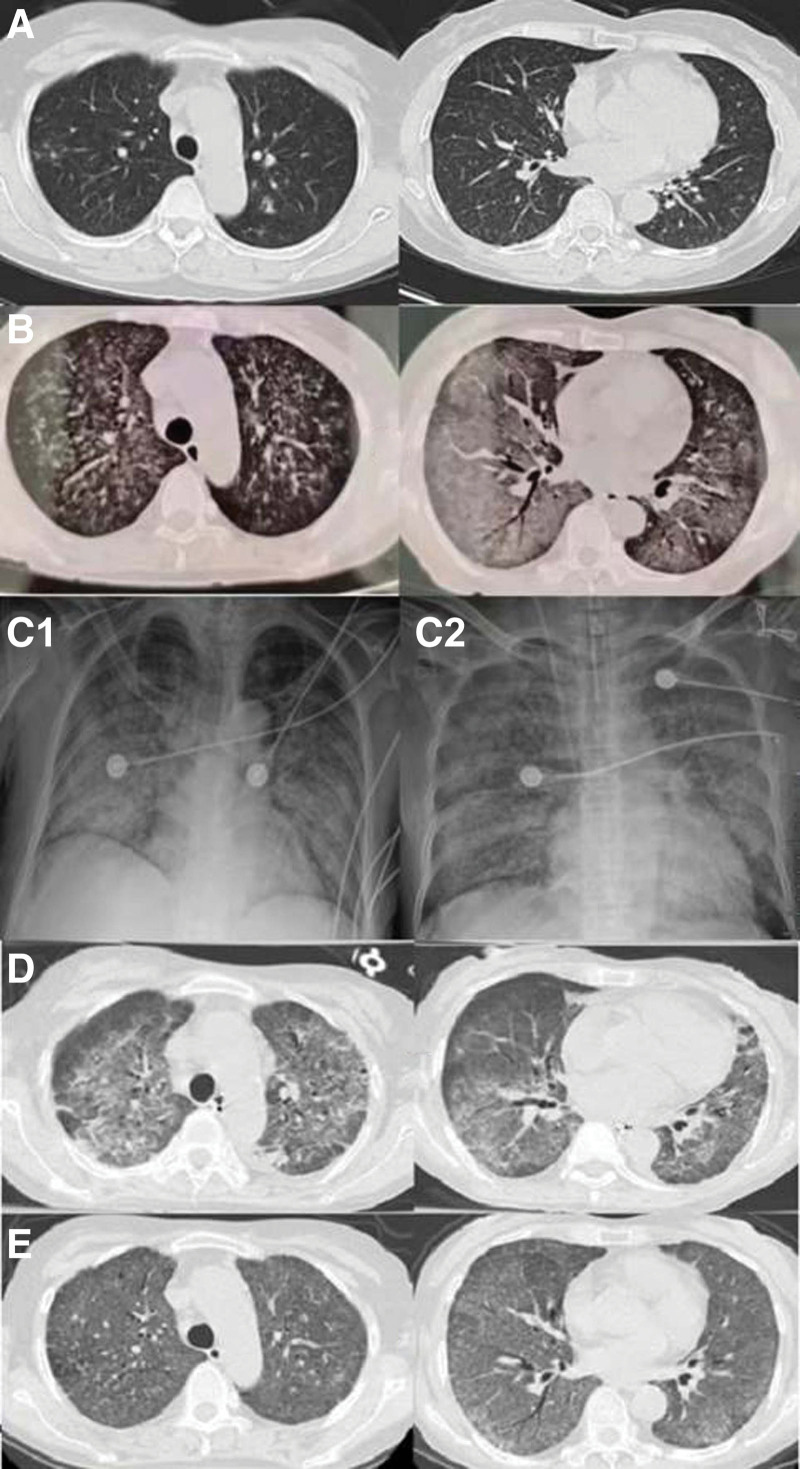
Chest computed tomography scan. (A) Bilateral pulmonary nodules during the first clinic visit. (B) Bilateral patchy infiltrations, consolidations, and ground-glass shadows 19 days after initiating antituberculosis therapy. (C) Right, at the intensive care unit admission, chest X-ray showed bilateral diffuse infiltrations. Left, after rifampin infusion on day 9, increased bilateral infiltrations. (D) Decreased bilateral lung infiltrations on day 20. (E) Improved bilateral lung infiltrations 1 month after the hospital discharge.
